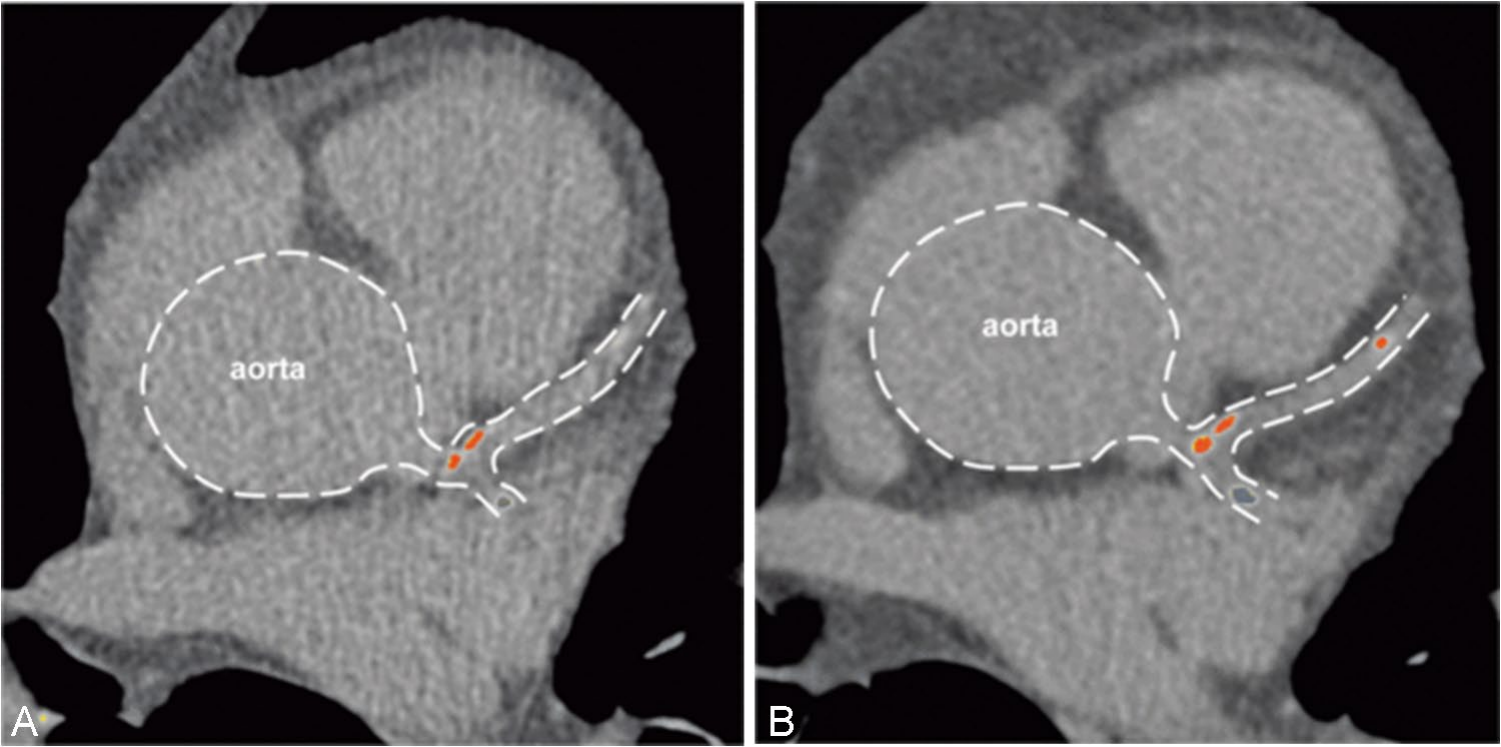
Progression of atherosclerosis in an SLE patient with no cardiovascular complaints at 1 year of follow-up. Multidetector CT calcium score examination. A Two calcified plaques are seen in the left anterior descending artery (red) and one calcified plaque in the circumflex artery (blue): plaque volume 156.4 mm^3^, calcium score 138.9. B After 1 year, the volume of the previously observed plaques increased with the new calcification in the distal part of the left anterior descending artery: plaque volume 223 mm^3^, calcium score 202.5
